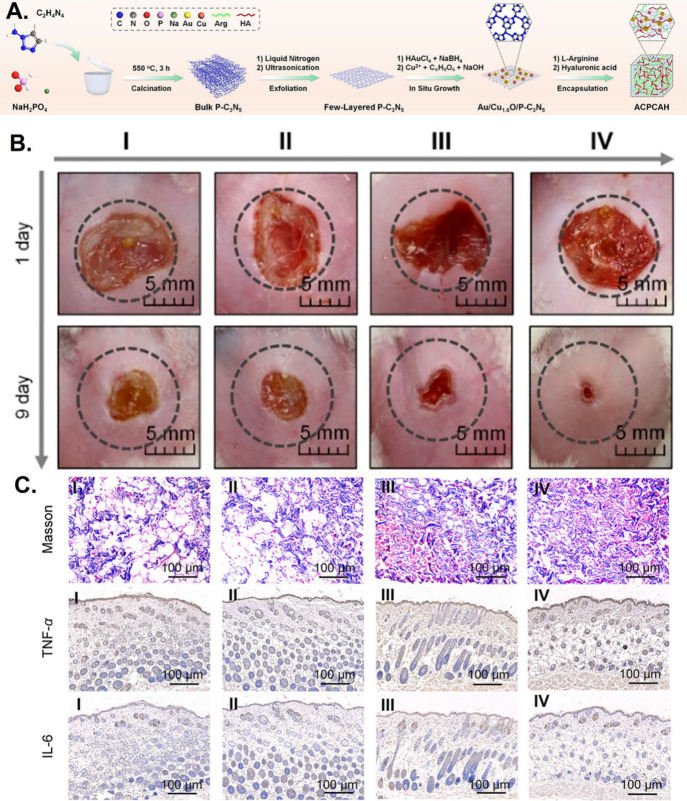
ACPCAH: Ultrasound-augmented
